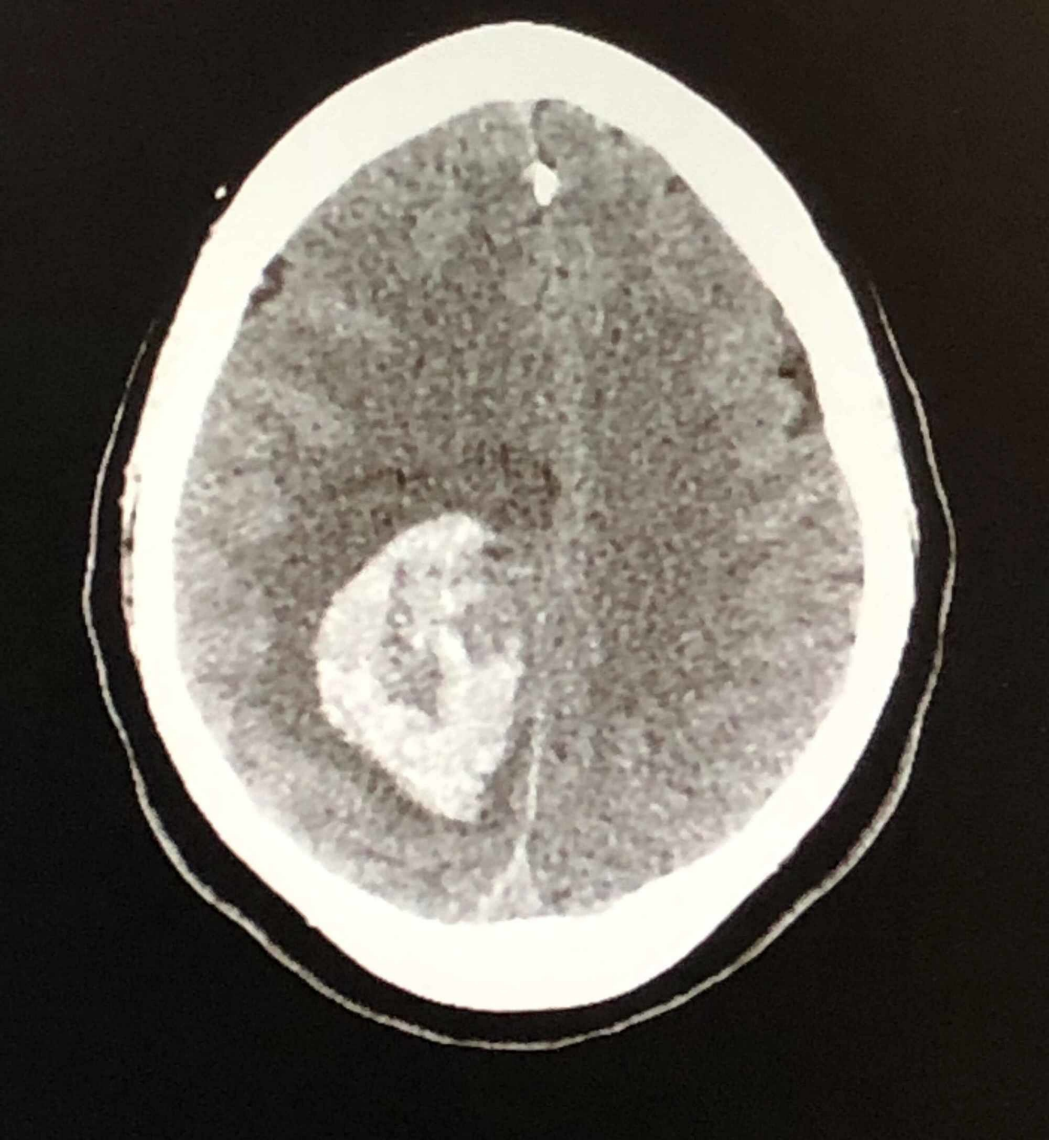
Non-contrast CT head Large acute hemorrhage in the right centrum semiovale measuring approximately 5.7 x 3.8 x 3.8 cm resulting in inferior displacement and effacement of the right lateral ventricle and approximately 8 mm of right-to-left midline shift.
